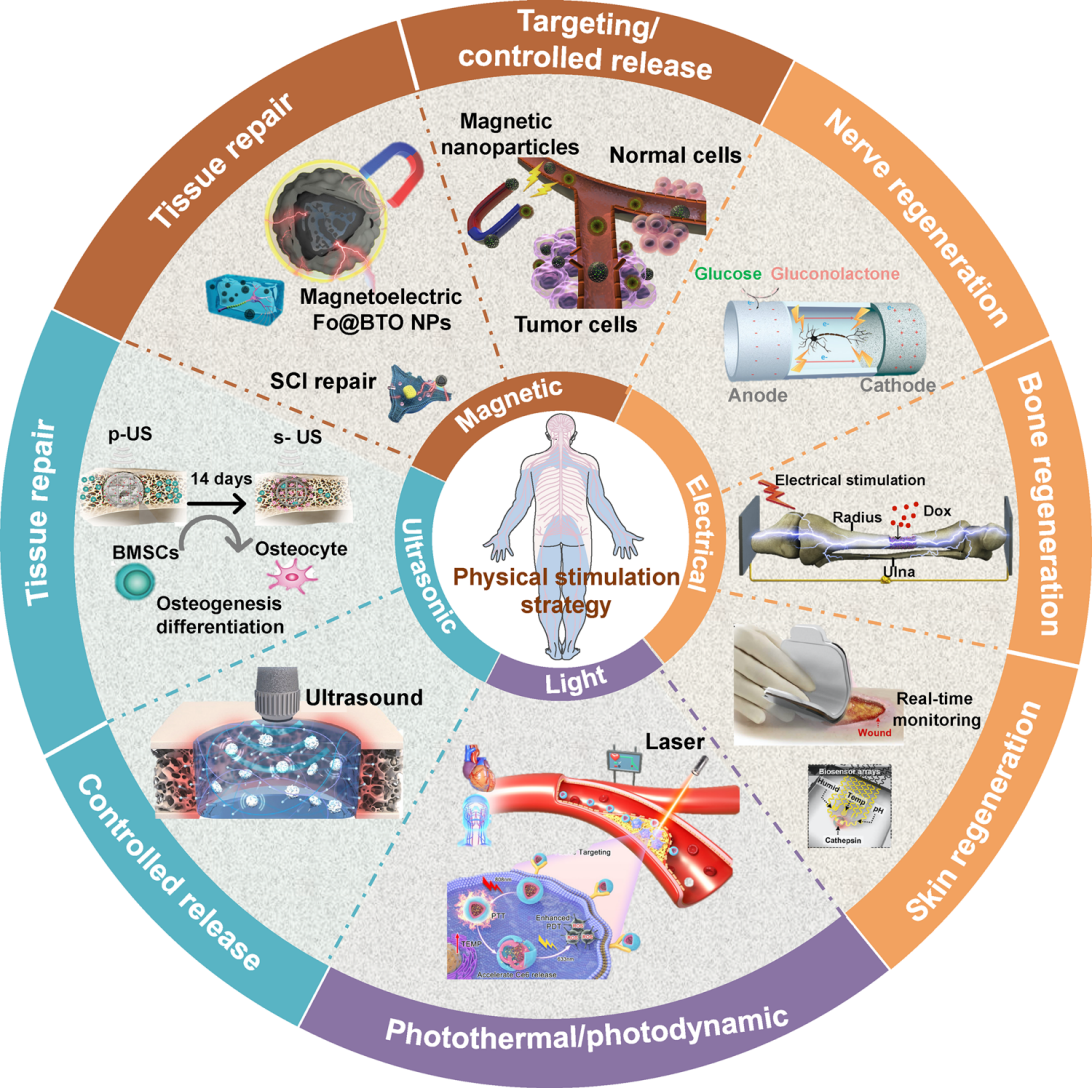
The application of physical stimulation biomaterials based on ultrasound, light, electricity, and magnetism in the field of biomedicine is summarized, as electrical stimulation of nerve regeneration. Reproduced with permission.^[^
